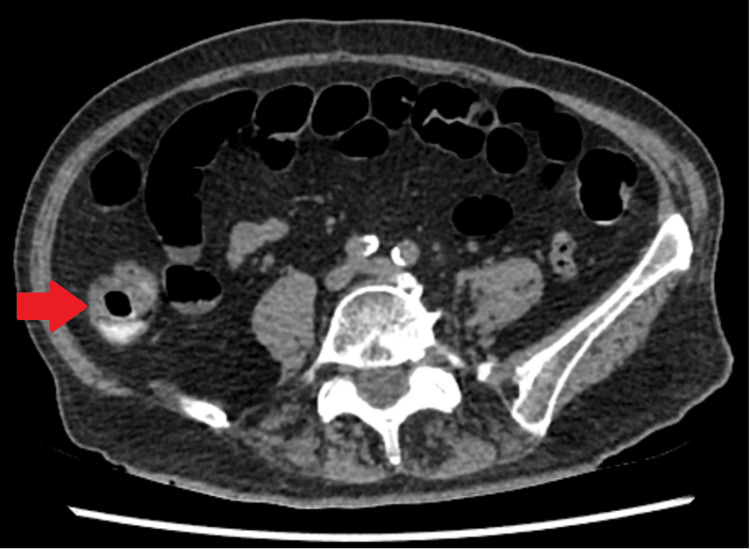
CTVC demonstrating ascending colonic thickening. CTVC: computed tomography virtual colonoscopy
